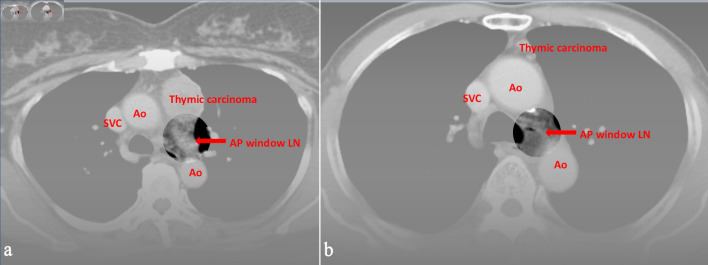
Preoperative CT images of (a) a 59-year-old female and (b) a 74-year-old male with World Health Organization (WHO) classification thymic carcinoma. All three radiologists evaluated LN metastasis in the aortopulmonary window, and the pathological report proved the positive findings. Ao: aorta; SVC: superior vena cava; CT: computed tomography; LN: lymph node.
